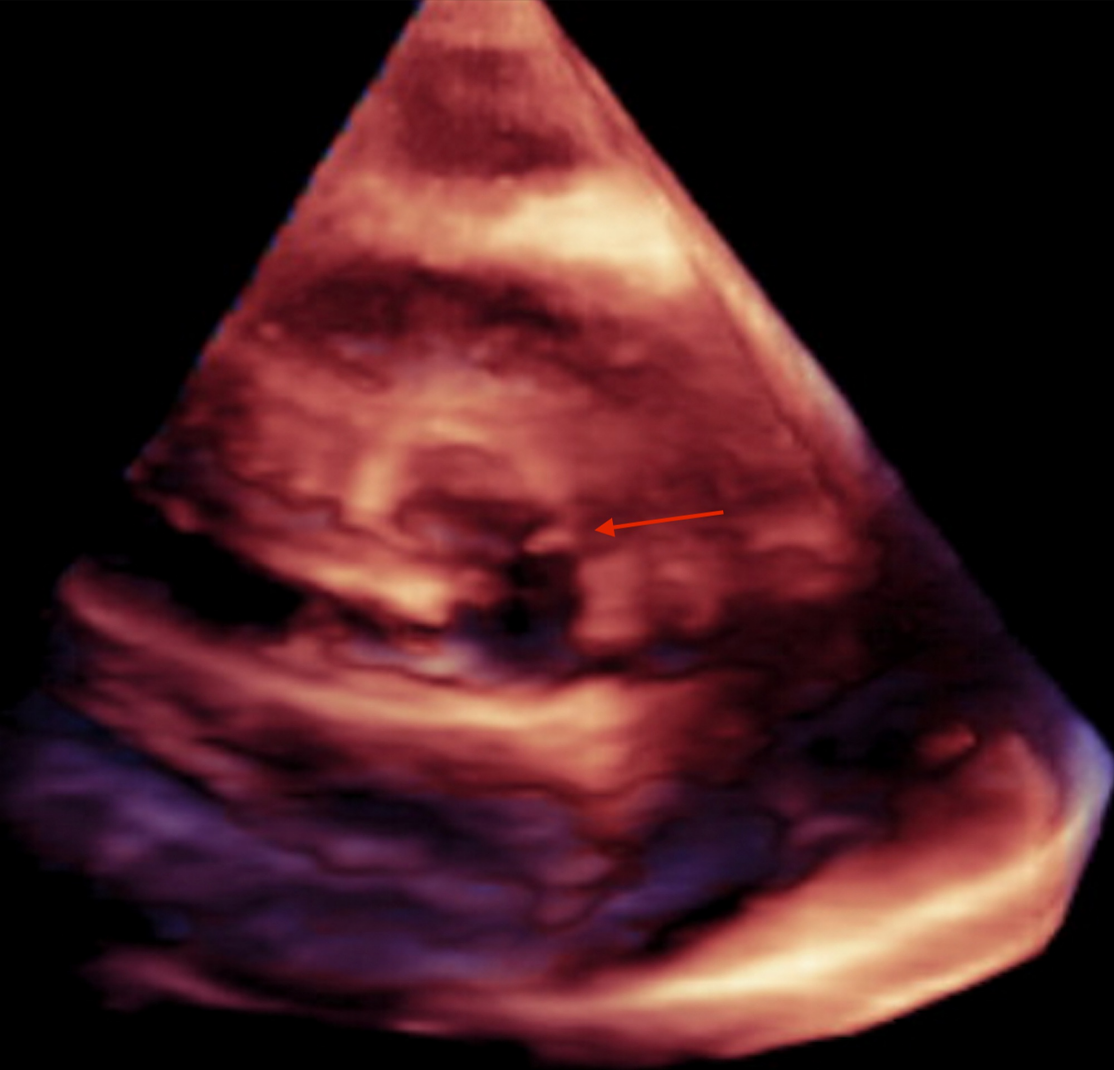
Aortic Valve Leaflet Laceration This is a three-dimensional echocardiographic image of the aortic valve with the arrow directed toward a tear of the left coronary leaflet.
